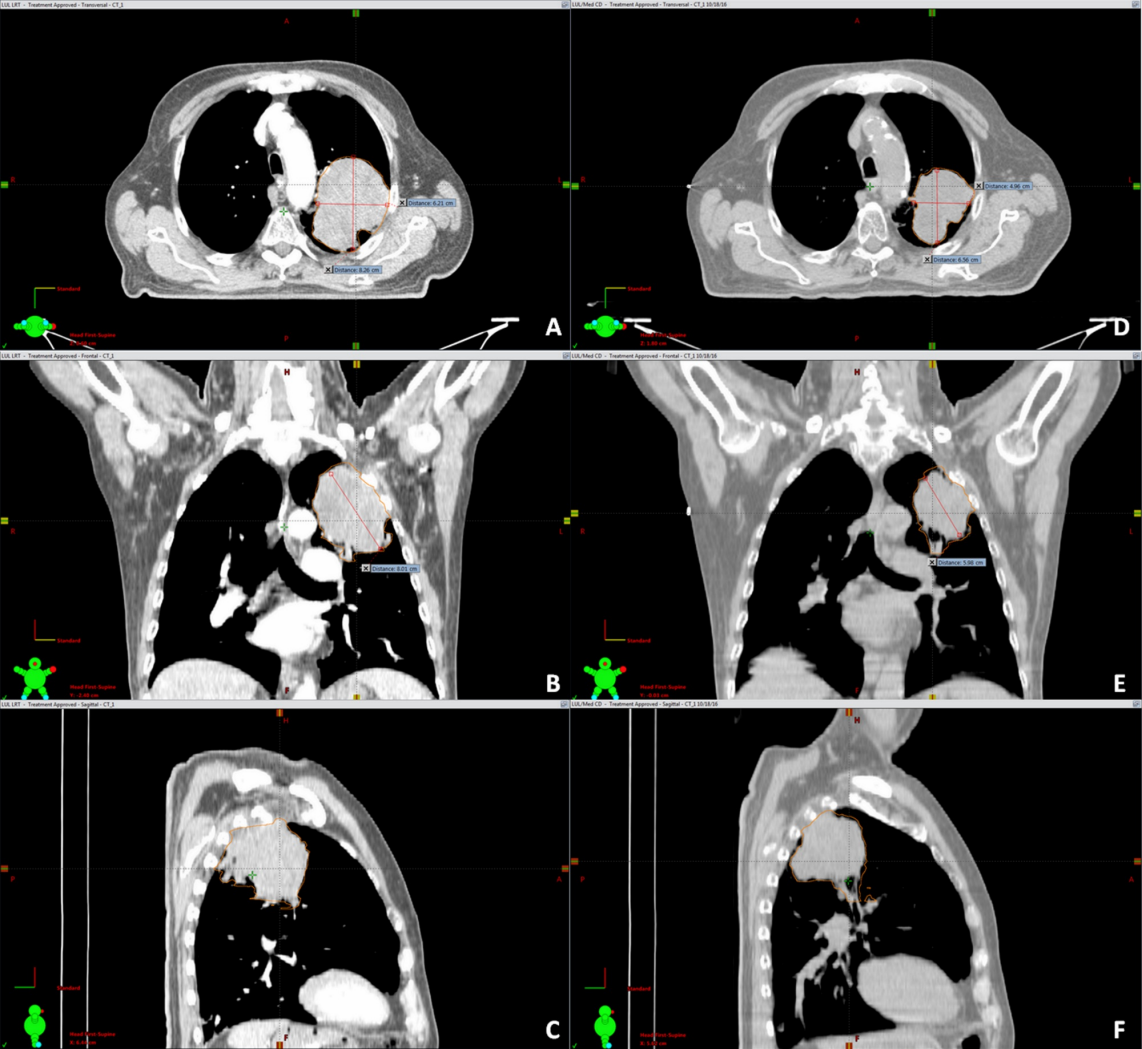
Significant decrease in tumor volume after completion of LRT followed by conventional XRT irradiation Significant decrease in volume after completion of lattice radiotherapy (LRT) followed by conventional irradiation in a 55-year-old male who presented initially with metastasis in the femur treated with radiation therapy. Following that, the primary non-small cell lung cancer (NSCLC) received LRT followed by conventional radiotherapy. After 18 months, the patient is alive with local control. Figures 2A, 2B, 2C (left): planning computed tomography (CT) scans in the axial (A), coronal (B) and sagittal views (C) before LRT with gross tumor volume (GTV) = 110.48 cc. Figures 2D, 2E, 2F (right): corresponding axial (D), coronal (E), and sagittal images (F) of the cone-beam CT (CBCT) obtained on the last day of treatment demonstrates a tumor reduction of 67% corresponding to a residual volume of 36.46 cc.
